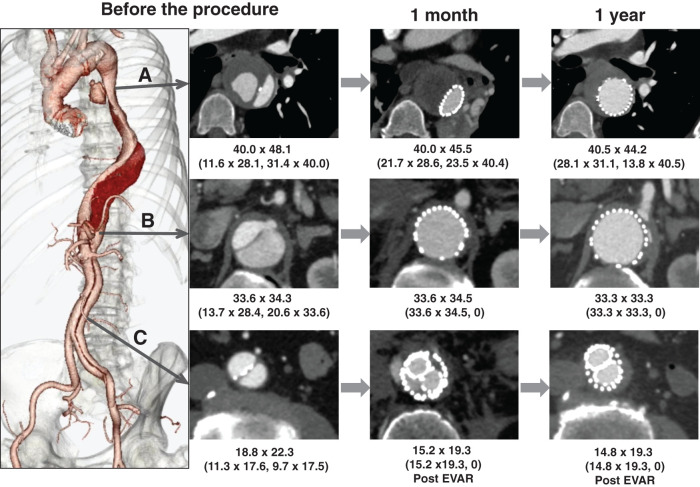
CT images of case 2 at 3 time points: preoperatively, 1 month, and 1 year after the STABILISE procedure. Aortic diameter (short axis × long axis, mm); TL and FL diameters are shown in parentheses. (A) Descending thoracic aorta at T4–T5 level. Preoperatively, aneurysm enlargement and retrograde flow from FL were observed in a previously thrombosed saccular aneurysm. At 1 month, FL was completely thrombosed. At 1 year, TL was dilated and FL had markedly decreased, with associated aneurysm shrinkage. (B) Abdominal aorta at the level of the celiac artery bifurcation. Preoperatively, FL was patent, and the celiac artery originated from compressed TL. At 1 month, TL was dilated and FL was obliterated; this was maintained at 1 year. (C) Abdominal aorta at the inferior mesenteric artery level. Preoperatively, FL was patent, and calcification was observed along the dissected intimal flap. Intraoperatively, aortic rupture occurred, and emergency EVAR was performed. At 1 month, FL had disappeared and the lumen outside EVAR limbs was thrombosed. At 1 year, the lumen outside EVAR had further decreased in size. FL: false lumen; TL: true lumen; STABILISE: stent-assisted balloon-induced intimal disruption and relamination in aortic dissection repair; CT: computed tomography; EVAR: endovascular aneurysm repair
